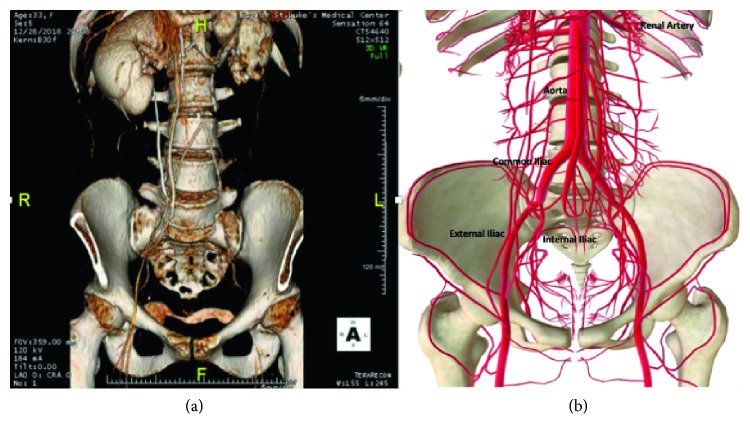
Computed tomography angiogram of the abdomen, pelvis, and lower extremities with runoff (a). Computer generated pelvic vasculature demonstrating the stark difference (b).
